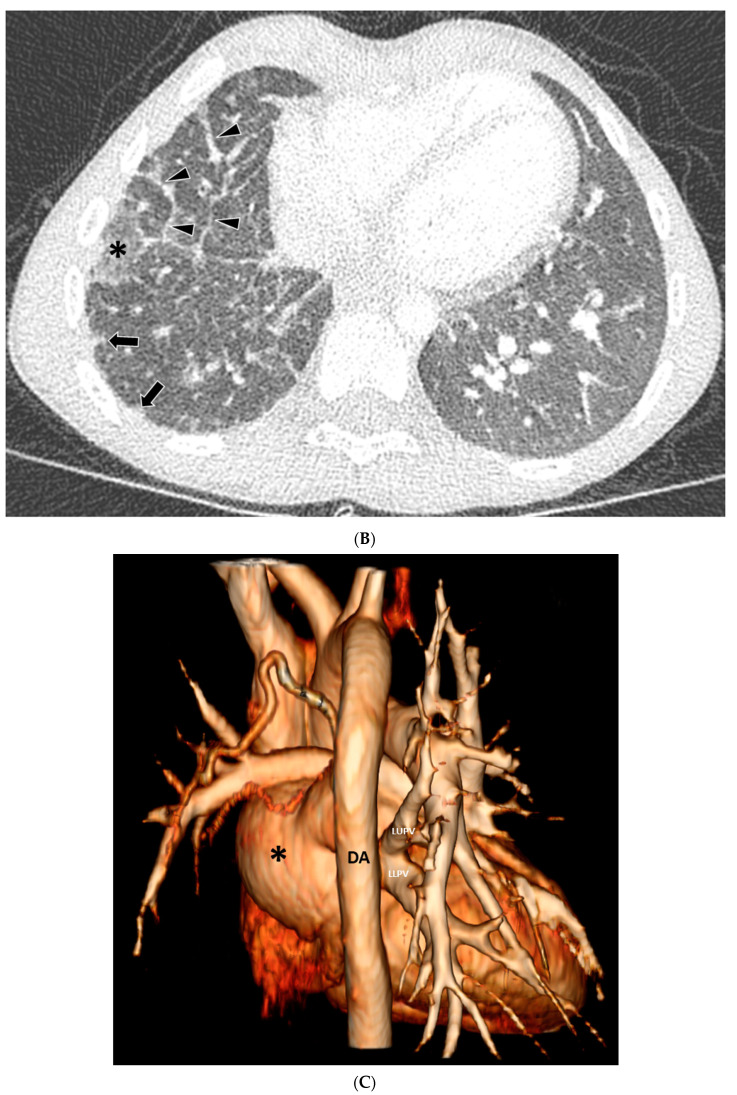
An 8-month-old male with secondary pulmonary vein stenosis due to total anomalous pulmonary venous connection repair who presented with shortness of breath. (A) Axial contrast-enhanced thoracic MDCT angiography CT image shows ill-defined, mildly heterogeneously enhancing, non-calcified soft tissue mass (asterisk) in the expected location of the right pulmonary vein. Right pulmonary vein is absent. Pleural thickening (arrow) is seen. Left upper pulmonary vein (LUPV) and left lower pulmonary vein (LLPV) are patent. LA = left atrium. (B) Axial lung window CT image demonstrates ground-glass opacity (asterisk) in the right lower lobe, septal thickening (arrowheads), and pleural thickening (arrows). (C) 3D volume-rendered vascular reconstruction CT image in the posterior projection view shows absent right pulmonary vein in the expected location (asterisk). Patent left upper pulmonary vein (LUPV) and left lower pulmonary vein (LLPV) are seen. DA = descending aorta.
